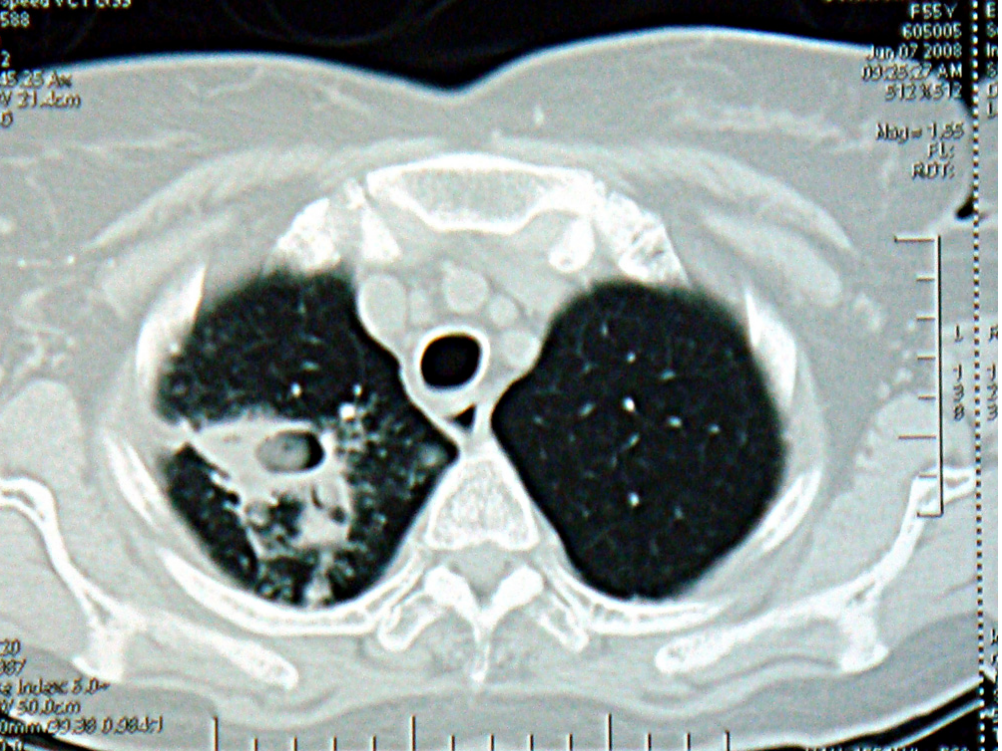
Computed tomogram of the thorax. Thick walled cavity in the right lung upper lobe with an indwelling aspergilloma.
